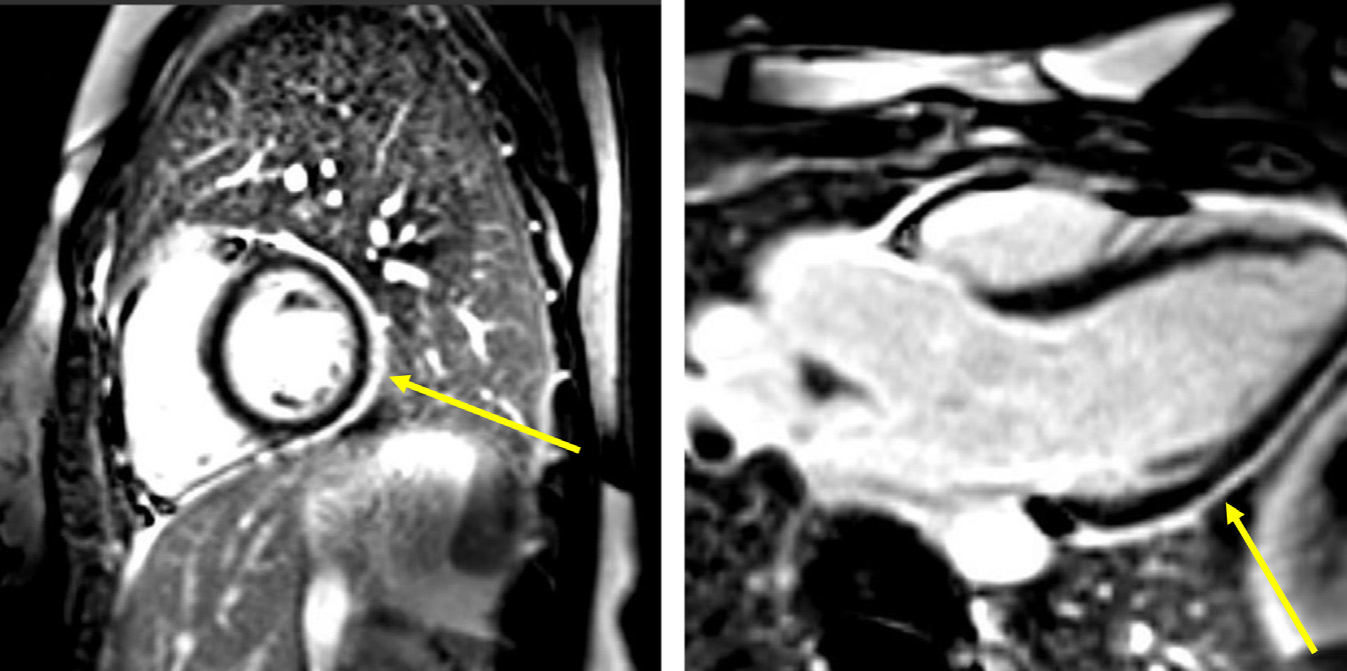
Cardiac Magnetic Resonance Imaging Short-axis (left) and 3-chamber (right) views of cardiac magnetic resonance imaging using a phase-sensitive inversion recovery sequence, showing circumferential pericardial late gadolinium enhancement (yellow arrow).
